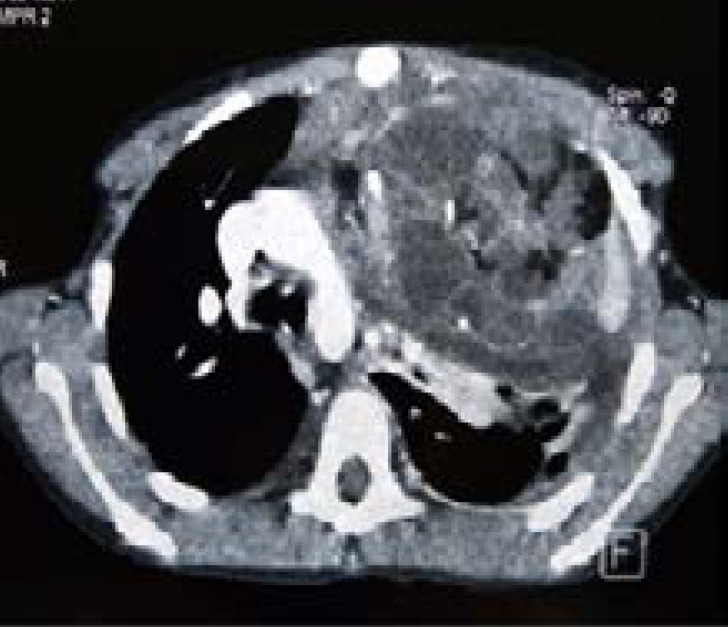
Chest CT showing heterogenous left-sided mass with many areas of calcifications
